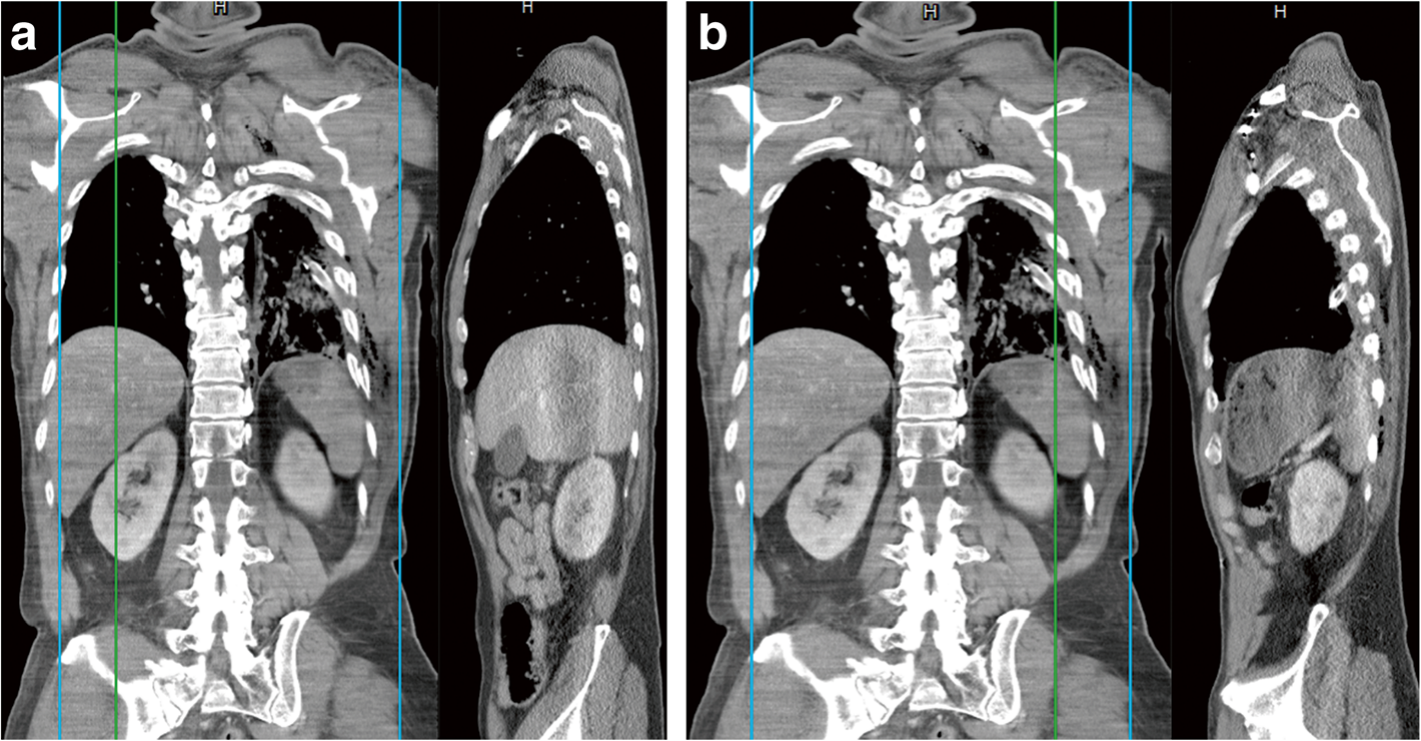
Computed tomography (CT) scan of the chest of Case no. 3 reveals normal dome shape of the right posterior diaphragm (a). Contralateral sagittal reconstruction of the CT scan of the chest reveals loss of the dome shape and altered contours of the left posterior diaphragm secondary to being trapped by the fractured left 9th and 10th ribs (b)
